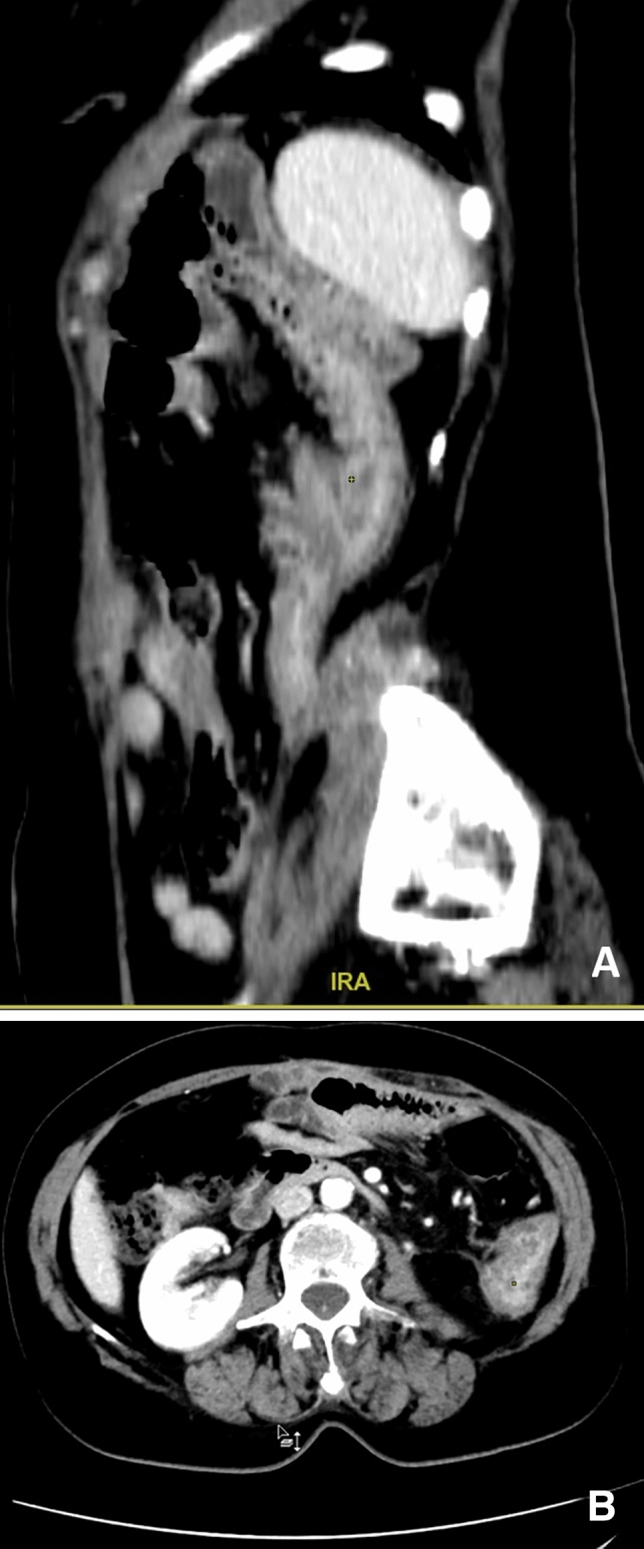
CT imaging in coronal and axial plane (a and b). Abdominal CT with IV iodinated contrast showed sigmoid diverticulitis with colonic wall thickening and pericolic fat stranding
